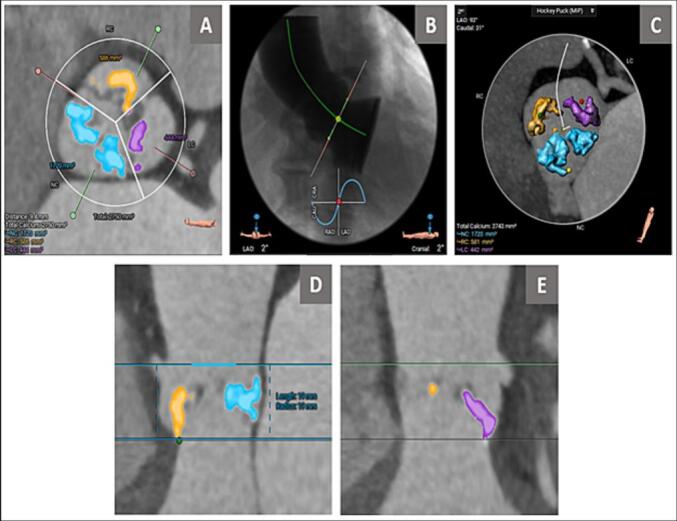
Example of one of our patients, Aortic valve calcification volume evaluation using 3Mensio CT software:
